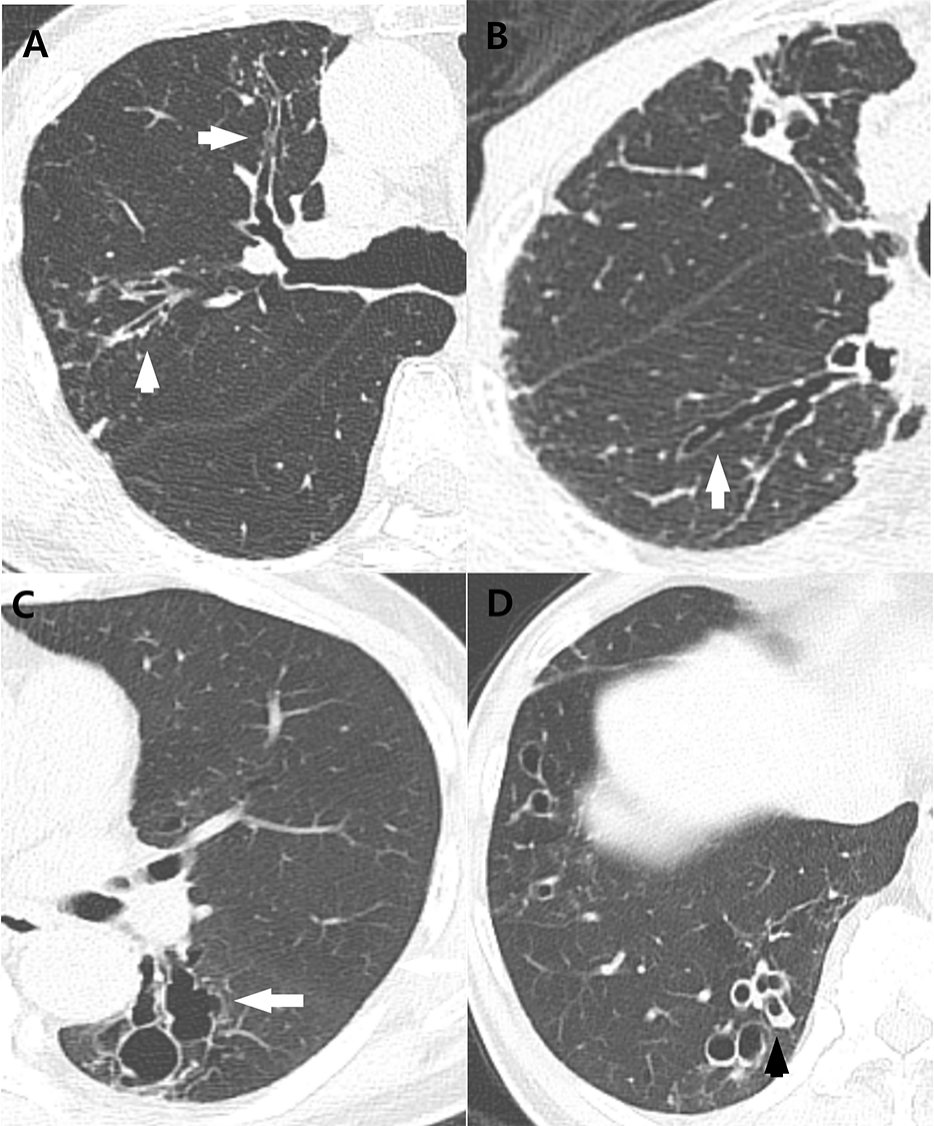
Examples of bronchiectasis to CT scoring of MAC lung disease. (A) Mild bronchiectasis in anterior and posterior segments of right upper lobe of 67-year-old man, scored as 1 point, with bronchus diameter greater than adjacent vessel diameter (arrows). (B) Moderate bronchiectasis in superior segment of right lower lobe of 86-year-old woman, scored as 2 points, with bronchus diameter two to three times vessel diameter (arrow). (C) Severe bronchiectasis in superior segment of left lower lobe of 66-year-old man, scored as 3 points, with bronchus diameter greater than three times vessel diameter (arrow). (D) Mucus plugging associated with severe bronchiectasis in posterior basal segment of right lower lobe of 66-year-old man (arrow).
